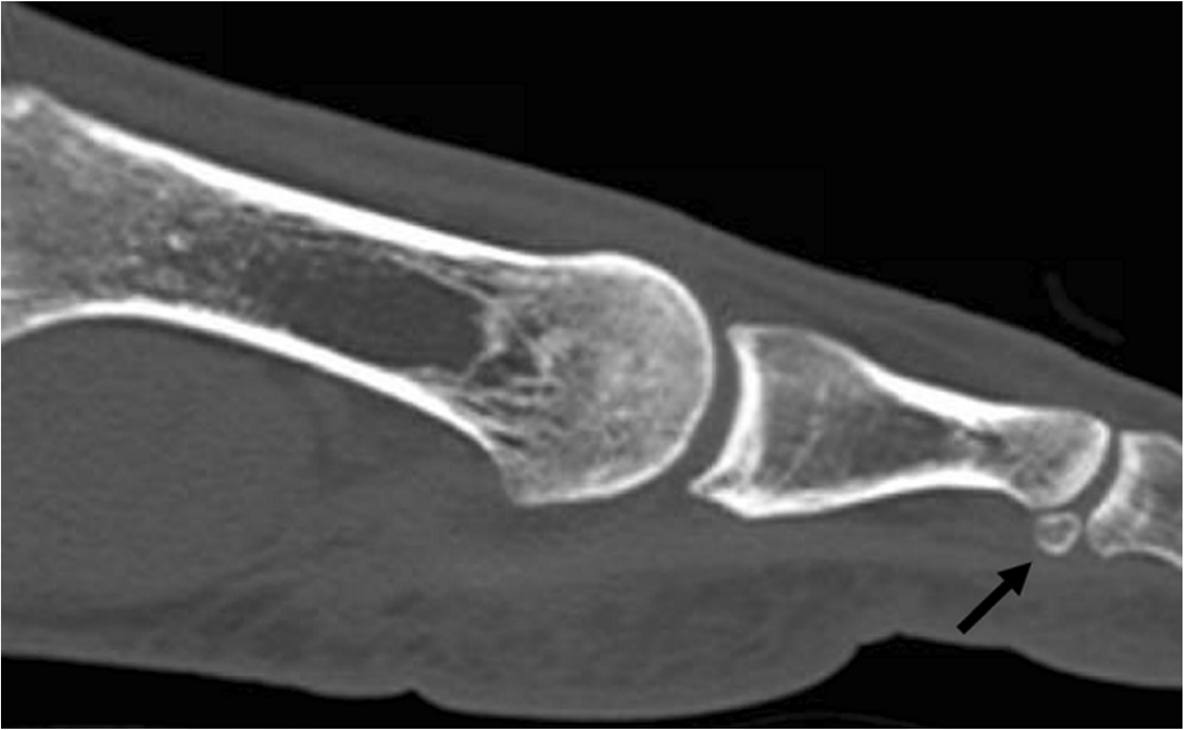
Interphalangeal joint sesamoid. A 33-year-old man referred for flat foot. Incidental finding of an interphalangeal joint sesamoid. Sagittal CT reconstruction better demonstrates its position with respect to the interphalangeal joint (black arrow). A potential complication arises if there is a phalangeal dislocation and the ossicle displaces into the joint space, blocking reduction
